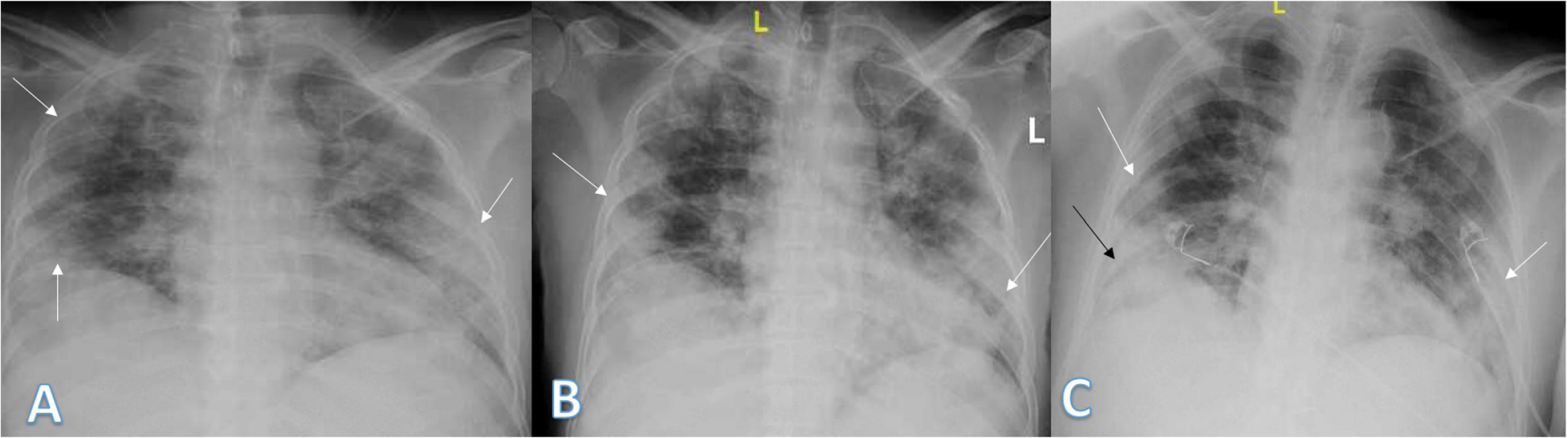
48-year male with positive COVID-19 virus. Initial chest X-ray (a) showed air space consolidation opacities at the periphery of the right lung and the mid and lower zones (arrows) with TSS as 4 (2 for each lung). On the 3rd day (b) and 6th day (c), air space consolidation opacities (arrows) were almost the same with mild right pleural effusion (black arrow on c)
